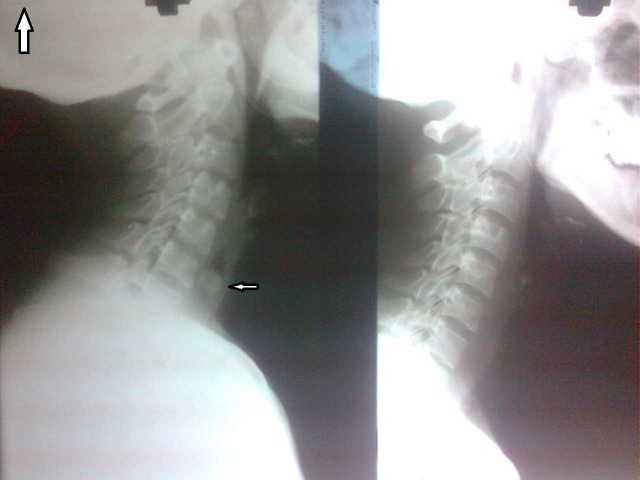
Postoperative radiograph showing normal cricopharynx
